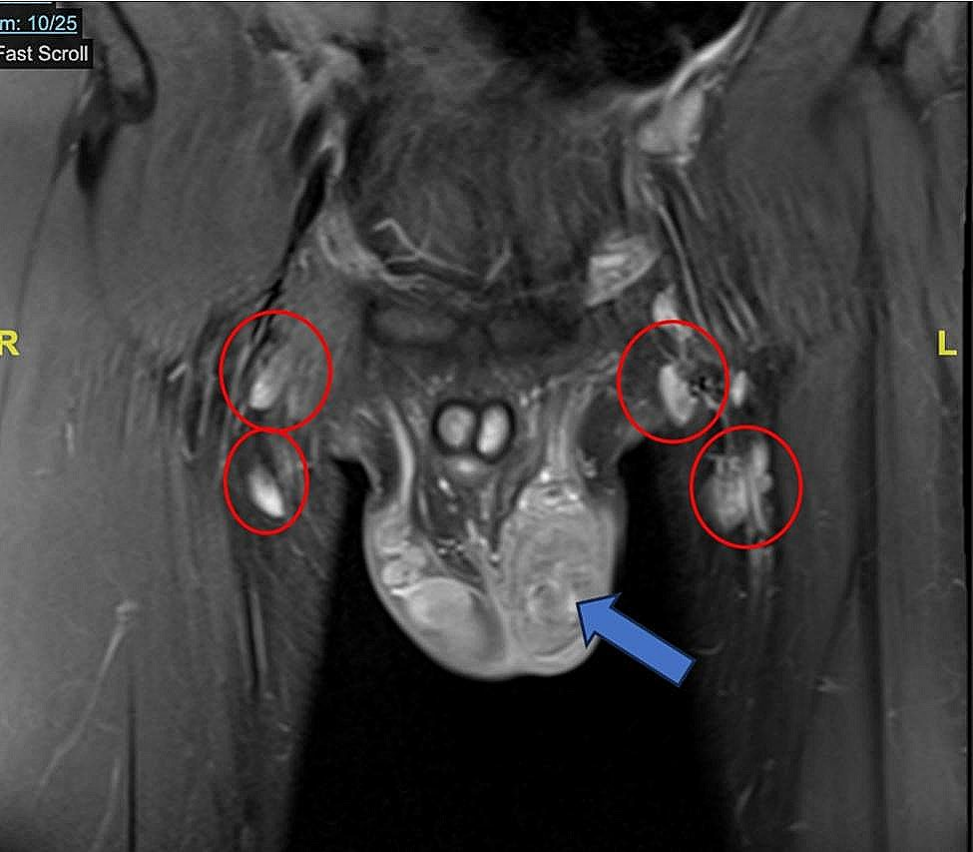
Genital MRI with contrast showed enlargement of epididymis. Post-contrast scanning provides inhomogeneous enhancement (Blue arrow) and enhancement of inguinal ring (Red circle)
